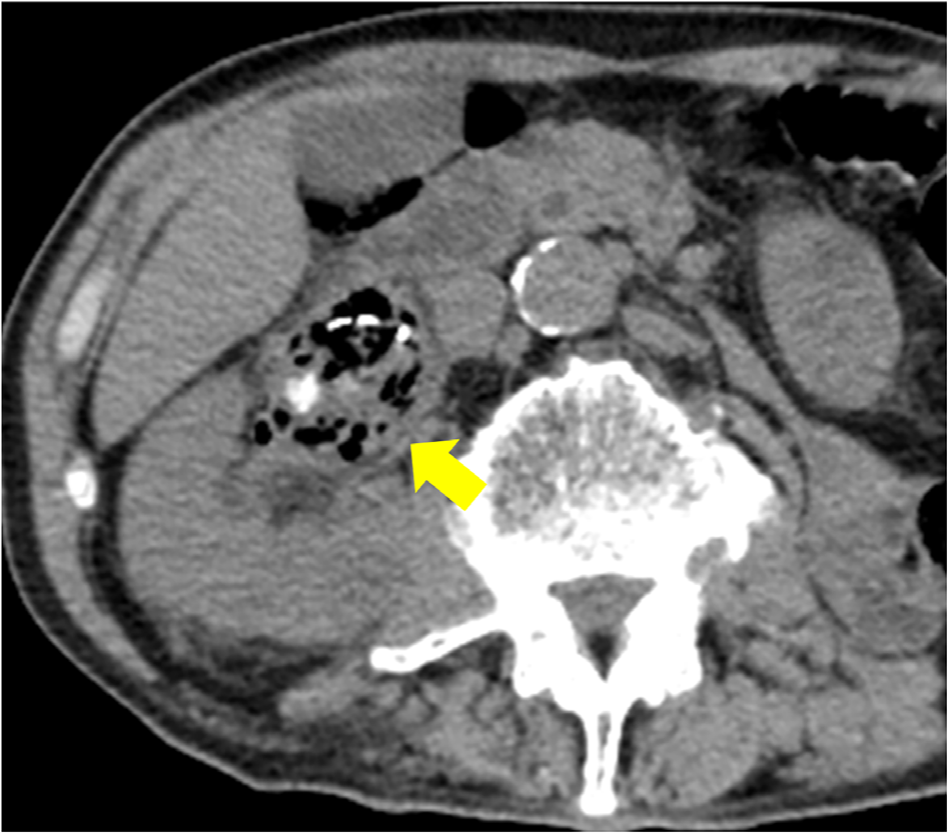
A mass that was separate with the renal pelvic wall, with air and calcification inside was, found within the renal pelvis. Because of the simple CT, it was difficult to assess the continuity between the renal pelvis wall and the mass.
